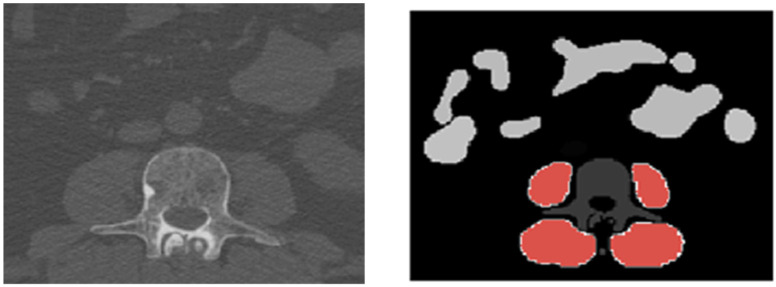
Example of muscle segmentation. (left) Axial view of whole-body low-dose CT without contrast agent at the level of the first lumbar vertebra. (right) AI-based segmentation of autochthone muscle and psoas muscle (red) with a total volume of 1149 cm^3^: vertebra in dark grey and bowels in light grey.
